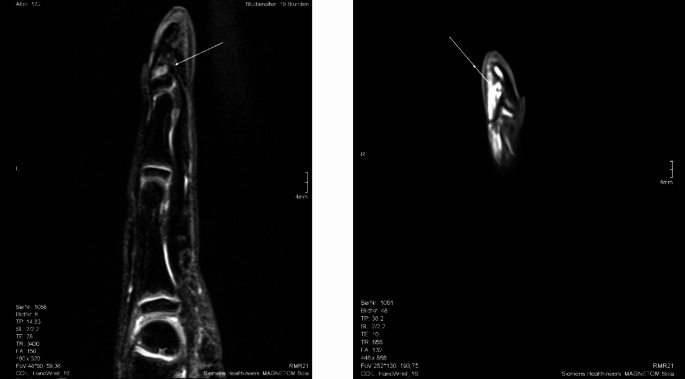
Preoperative MRI with a distally inserted flexor tendon without evidence of inflammatory activity in the epiphyseal area
